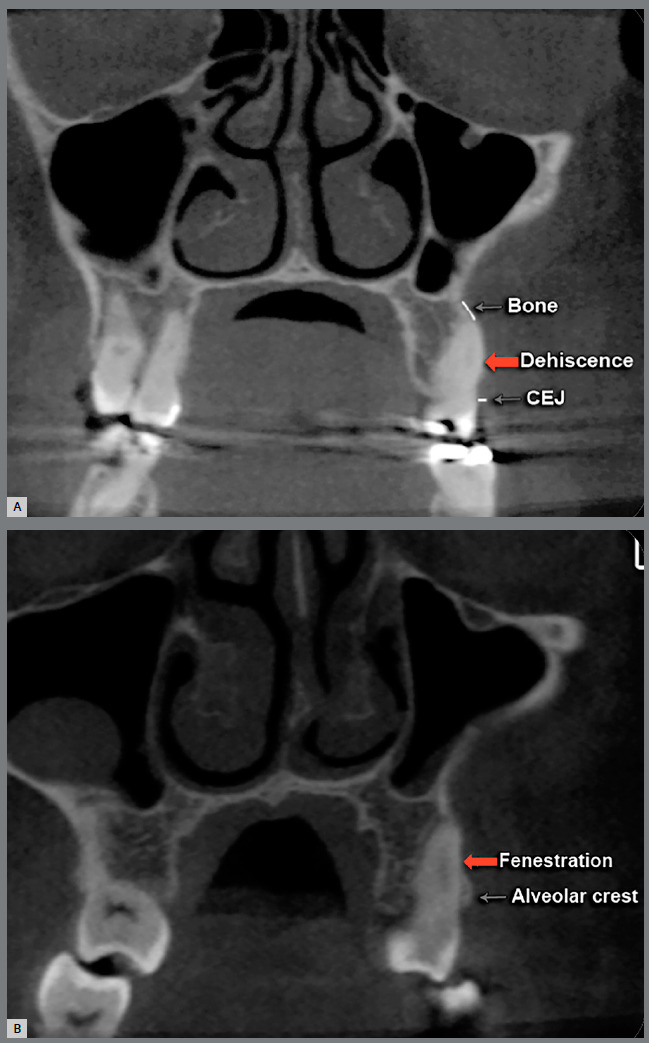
CBCT coronal slice showing dehiscence (A) and fenestration (B).
